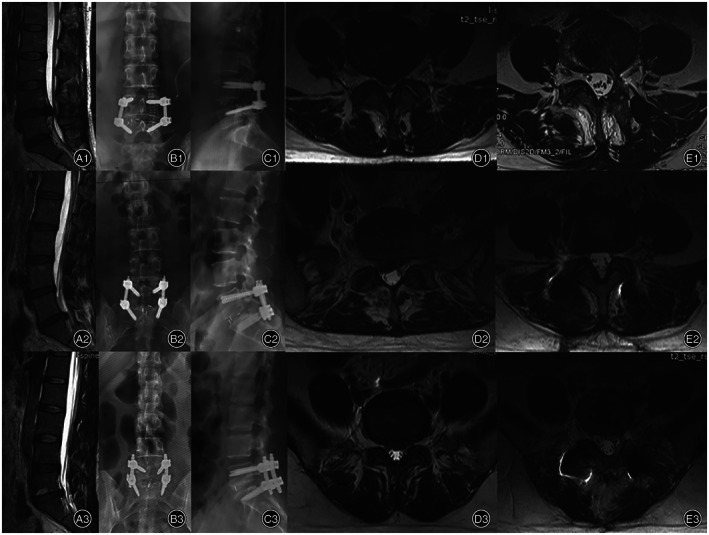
(A1–E1) One case of Wiltse approach TLIF patient. (A2–E2) One case of MIS‐TLIF patient, (A3–E3) One case of PLIF patient. (A1) MRI before surgery showed disc herniation in L4‐L5 segment. (B1 and C1) Postoperative anterior and lateral DR. (D1) Axial T2 MRI show disc herniation and multifidus before surgery. (E1) Axial T2 MRI show surgery level multifidus at last follow‐up after surgery with no significant atrophy and fatty infiltration. (A2) MRI before surgery showed disc herniation in L5‐S1 segment. (B2 and C2) postoperative anterior and lateral DR. (D2) Axial T2 MRI show disc herniation and multifidus before surgery. (E2) Axial T2 MRI show surgery level multifidus at last follow‐up after surgery with no significant atrophy and fatty infiltration. (A3) MRI before surgery showed disc herniation in L5‐S1 segment. (B3 and C3) Postoperative anterior and lateral DR. (D3) Axial T2 MRI show disc herniation and multifidus before surgery. (E3) Axial T2 MRI show surgery level multifidus at last follow‐up after surgery with significant atrophy and fatty infiltration.
